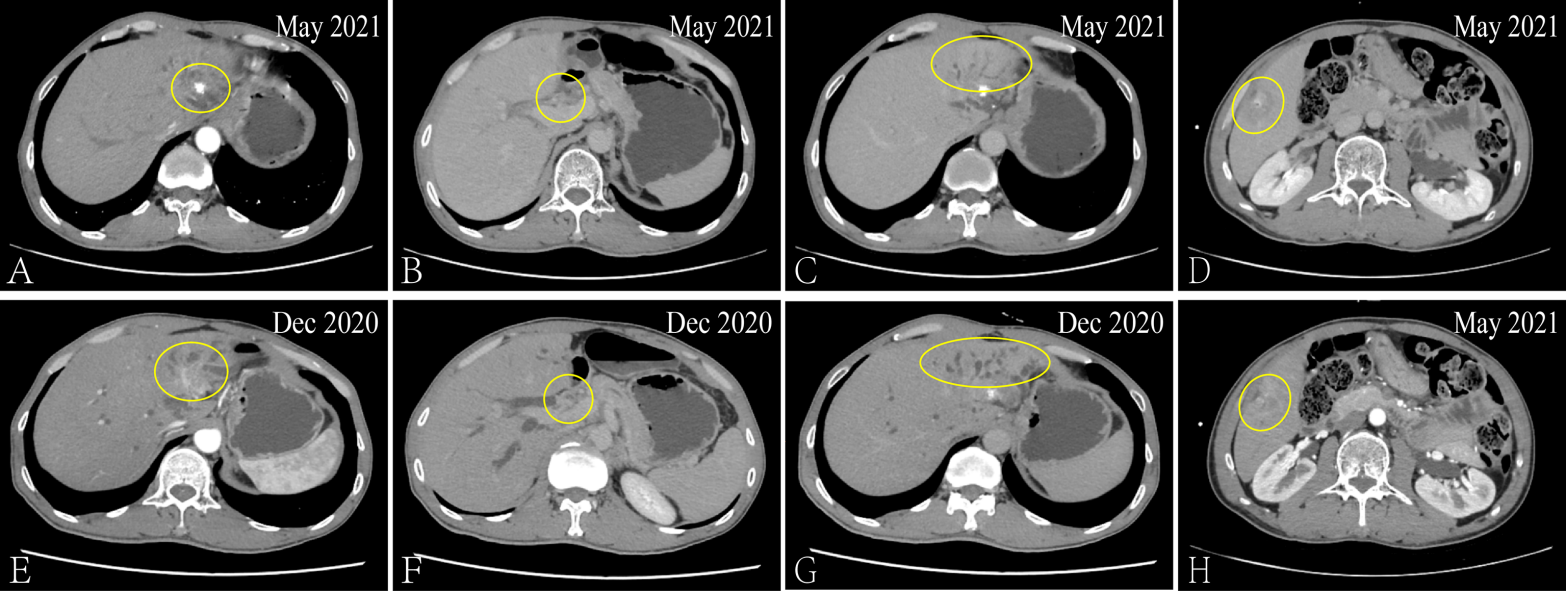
Via* CT to contrast the curative effect between the May 2021 and Dec 2020. The size of the metastatic lesion which in the left and caudate lobe of liver is reduction from 3.4 × 2.6cm to 2.6 × 2.1cm (A, E). The hepatic portal metastatic nodule has disappeared (B, F) and the compressive bile duct has improvement (C, G). The new metastatic lesion (2.7 × 1.7cm) in liver S5 (D, H).
